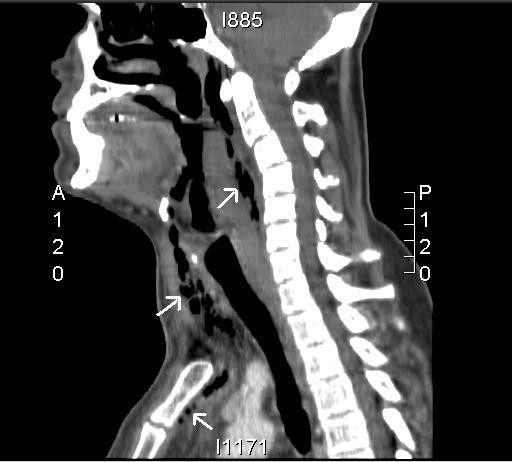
Sagittal reconstruction of CT of the neck and upper mediastinum; extensive soft tissue abnormality (swelling and surgical emphysema) in the retropharynx with loss of normal fat plane with the prevertebral space and possible communication with the oropharynx. Air (arrows) is also present in the pretracheal tissue and extends into the upper mediastinum.
